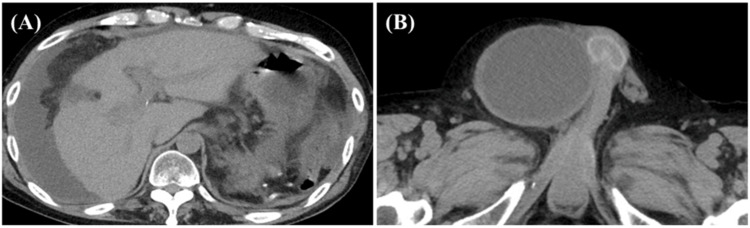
Computed tomography (CT) images at the time of admission (A) The liver exhibited an irregular surface with a large volume of ascitic fluid, along with increased density of intraperitoneal fat strands. CT revealed no evidence of intestinal perforation or other localized intra-abdominal infection. (B) A communicating right scrotal hydrocele was suspected.
